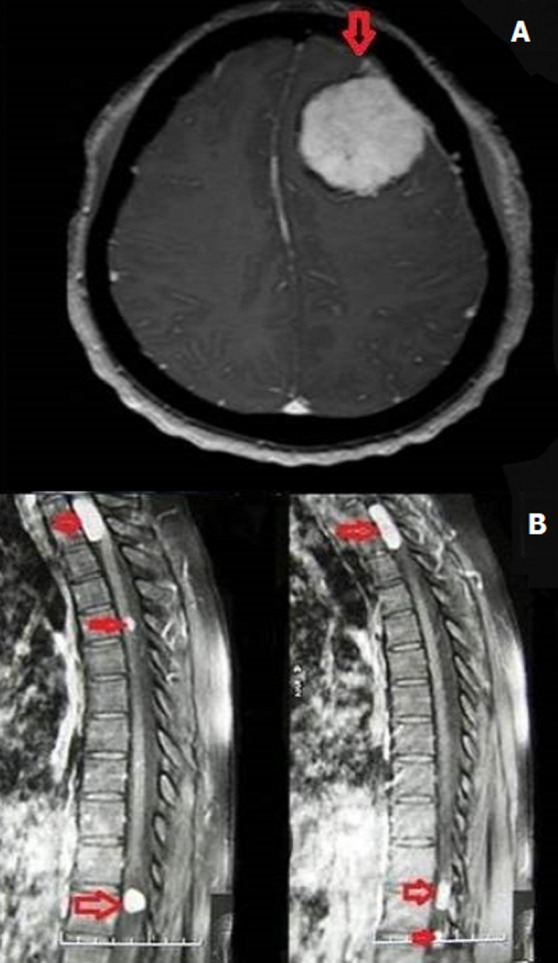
(A,B) brain MRI with contrast axial section showing frontal convexity meningioma, spine MRI on sagittal sections on T1 injection showing 4 lesions, the most compressive meningiomas are projected in T2 whose major axis is 28 mm
